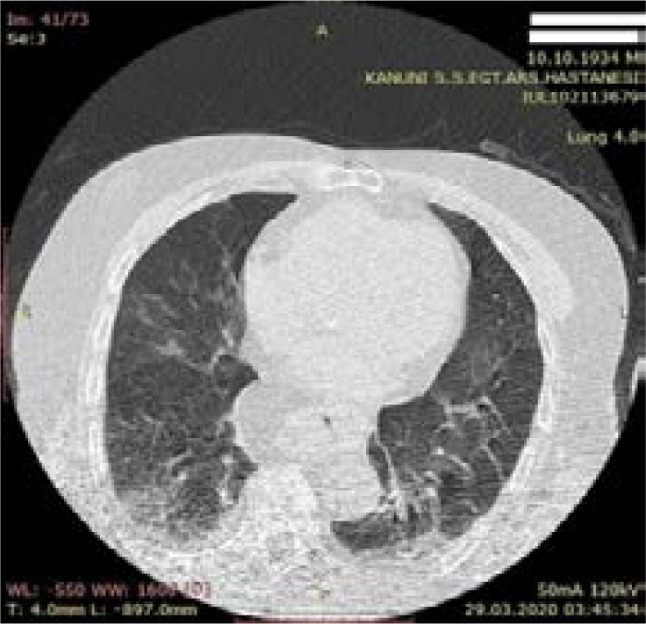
Typical mild CT finding - 85-year-old male patient - Dead - peripheral ground glass density in the lower lobe of both lungs
